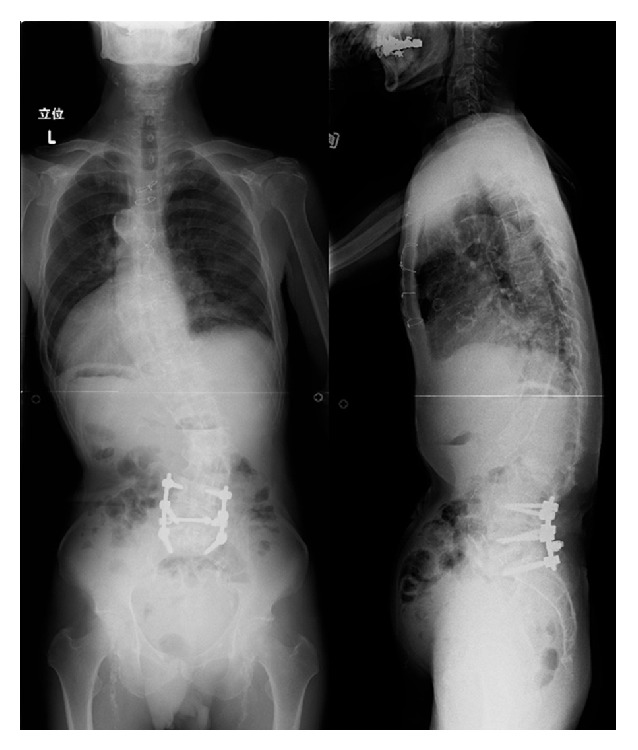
Postoperative radiographs showing coronal imbalance and pelvic retroversion that improved after surgery.
